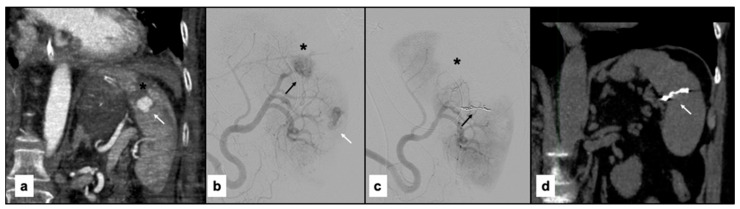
(a) Contrast-enhanced paracoronal CT scan acquired during the arterial phase shows a large laceration of the superior splenic pole (black asterisk) associated with a sizable pseudoaneurysm (arrow). (b) Digital subtraction angiography (DSA) performed using a 5F Cobra-shaped catheter (Cordis, FL, USA) confirms the laceration of the superior splenic pole (black asterisk) and the presence of the pseudoaneurysm (black arrow) previously identified on CT, with heterogeneous intraparenchymal vascularization (white arrow). (c) Post-procedural DSA performed through a guiding catheter demonstrates embolization of a branch feeding the vascular lesion using Penumbra coils (Crossmed, Turin, Italy) (black arrow), resulting in marked devascularization of the splenic parenchyma (black asterisk). (d) Follow-up non-contrast paracoronal CT reconstruction shows partial recovery of splenic volume in the area treated with the coils (white arrow).
